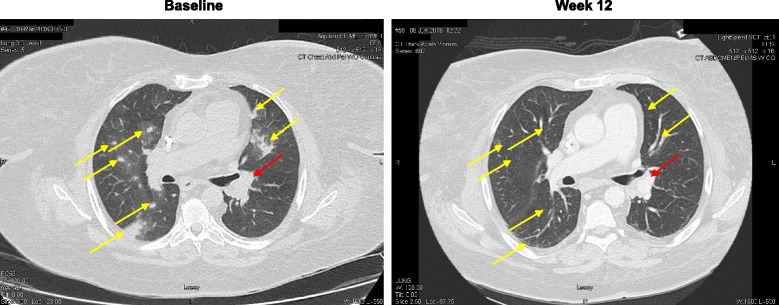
Radiographic response to nivolumab on hemodialysis; CT chest exams performed at baseline and 12 weeks after re-initiation of therapy demonstrates resolution of lung nodules (indicated by the *yellow arrows*). The hilar lymph node indicated by the *red arrow* is stable
